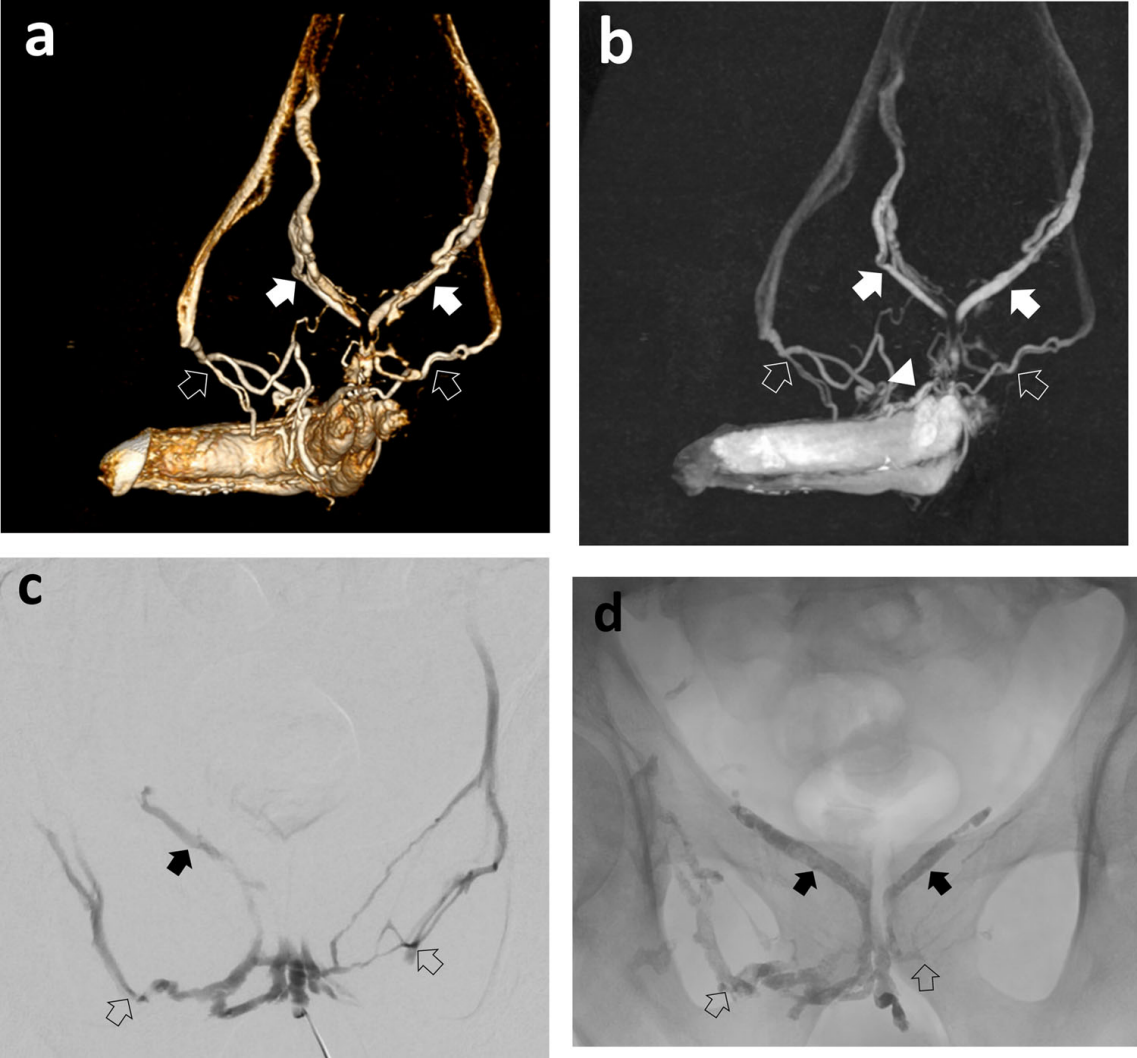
58-year-old patient with erectile dysfunction due to venous leak. Venographic depiction of a venous leak of internal (arrows) and external (open arrows) pudendal veins and large-caliber deep dorsal penile vein (arrowhead) on a CT cavernosography (volume rendering), b CT cavernosography (maximum intensity projection), c invasive venogram post direct puncture of the deep dorsal vein followed by embolization of large efferent veins using Glubran-2 d. His total IIEF-15 score improved from 20 at baseline to 67 at 6 weeks
